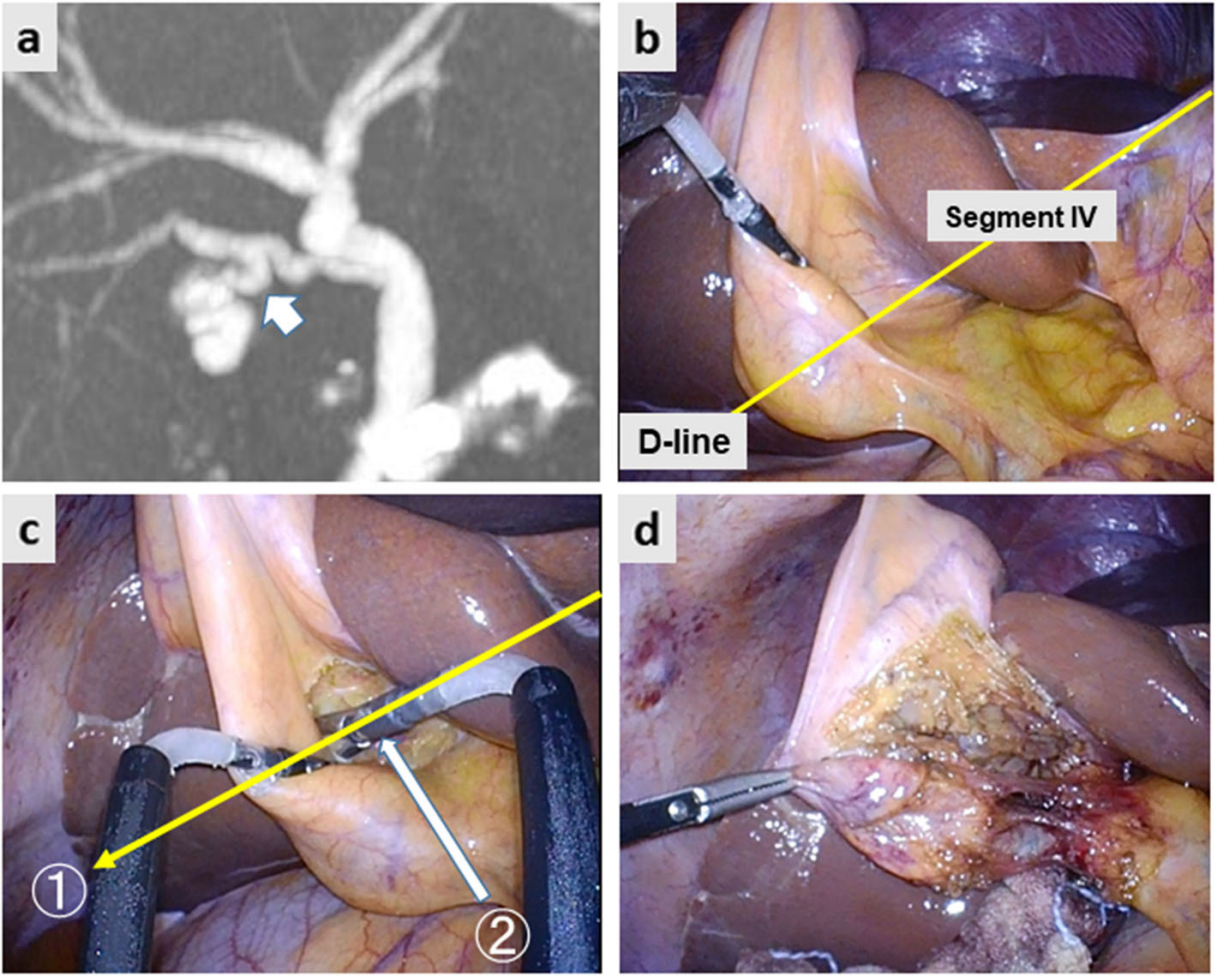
a Preoperative drip infusion cholangiography CT image. The white arrow indicates the cystic duct branched off the aberrant posterior sectional duct. b The imaginary diagonal line (D-line) of the segment IV of the liver (yellow arrow) was identified. c. Dissection of the gallbladder proceeded along the D-line (yellow arrow). The cystic structure was dissected towards the D-line (white arrow). d The critical view of safety was achieved
